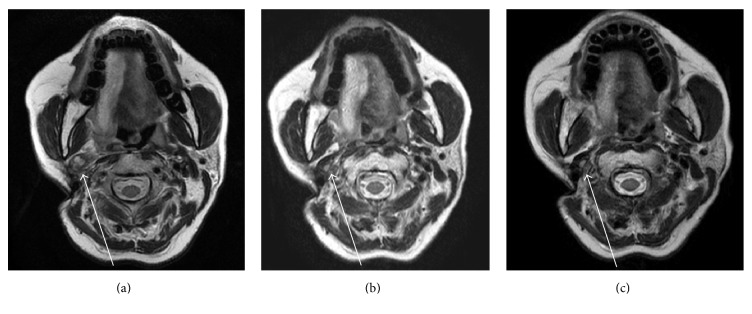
Magnetic resonance T2-weighted imaging findings. (a) Postsurgical status: a recurrent lymph node metastasis from a papillary thyroid carcinoma in the right parapharyngeal space is shown (white arrow). (b) Three months after stereotactic radiation therapy (SRT) using the CyberKnife radiotherapy system, the lymph node metastasis is remarkably reduced. (c) Twelve months after SRT, the reduced lymph node metastasis has not changed. No recurrences were recognized, which indicates therapeutic effectiveness.
